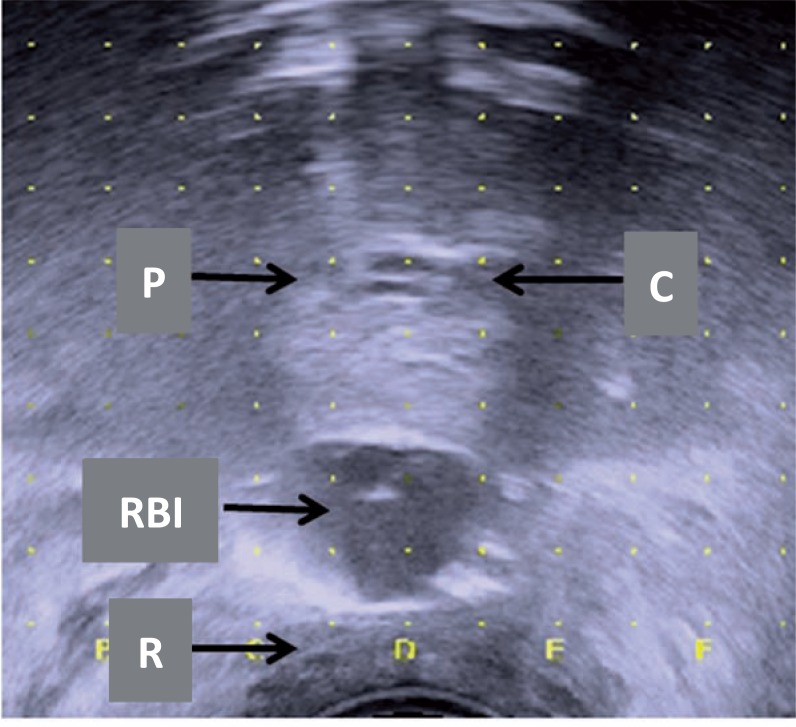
Axial ultrasound image: rectum balloon implant (RBI) being filled with saline between the prostate (p) and the rectum (R). note the urinary catheter (c) in the central plane, or ‘D-line’.
